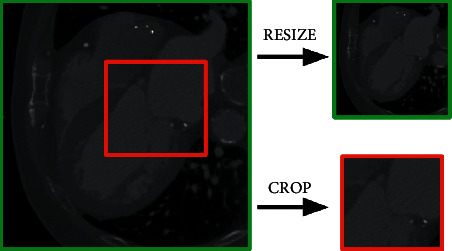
CT data can be resized (top right) or cropped (bottom right) depending on the application at hand. Resizing keeps the entire data but in a smaller format. Cropping takes out a region of interest without any resizing.
